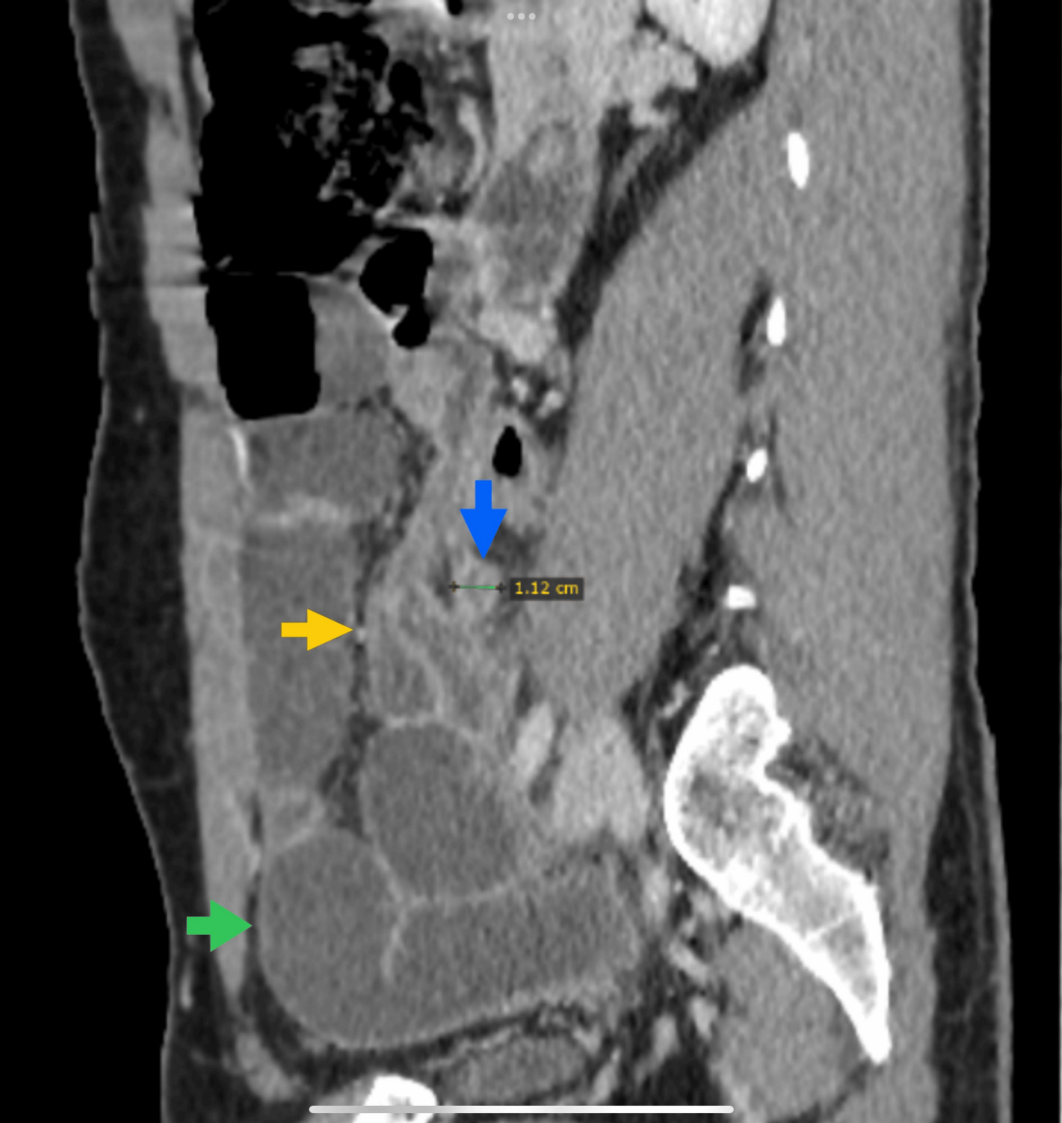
Sagittal contrast-enhanced CT image showing multiple dilated, fluid-filled small bowel loops (green arrow) with a transition point at the terminal ileum. The appendix is enlarged and hyperenhancing (1.12 cm, blue arrow) with surrounding peri-appendiceal inflammatory changes (yellow arrow).
